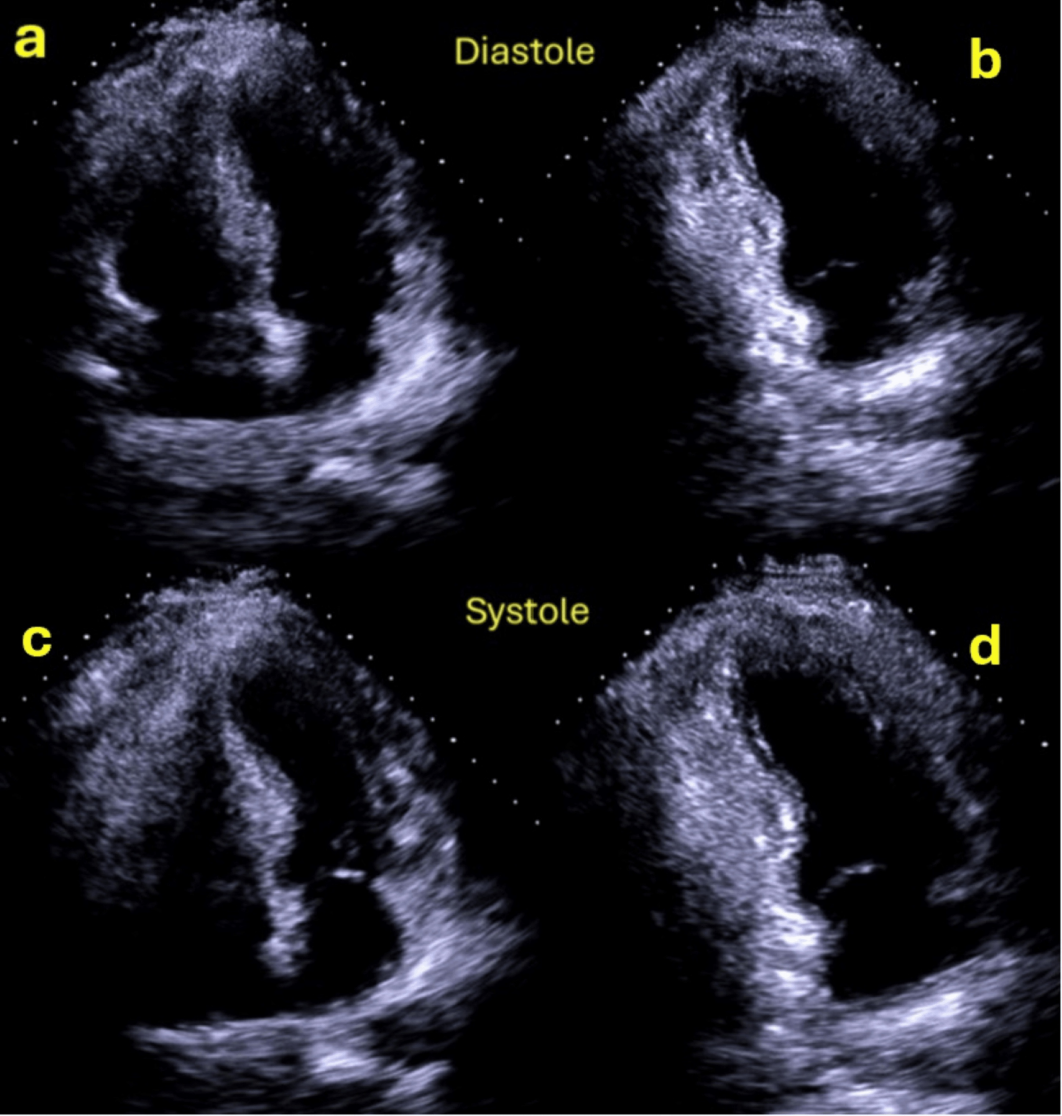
Echocardiogram performed at discharge showing improvement in segmental contractility alterations a: LV diastole apical four-chamber view; b: LV diastole apical two-chamber view; c: LV systole apical four-chamber view revealing improvement in segmental contractility alterations; d: LV systole apical two-chamber view also showing improvement in segmental contractility alterations LV: Left ventricle
